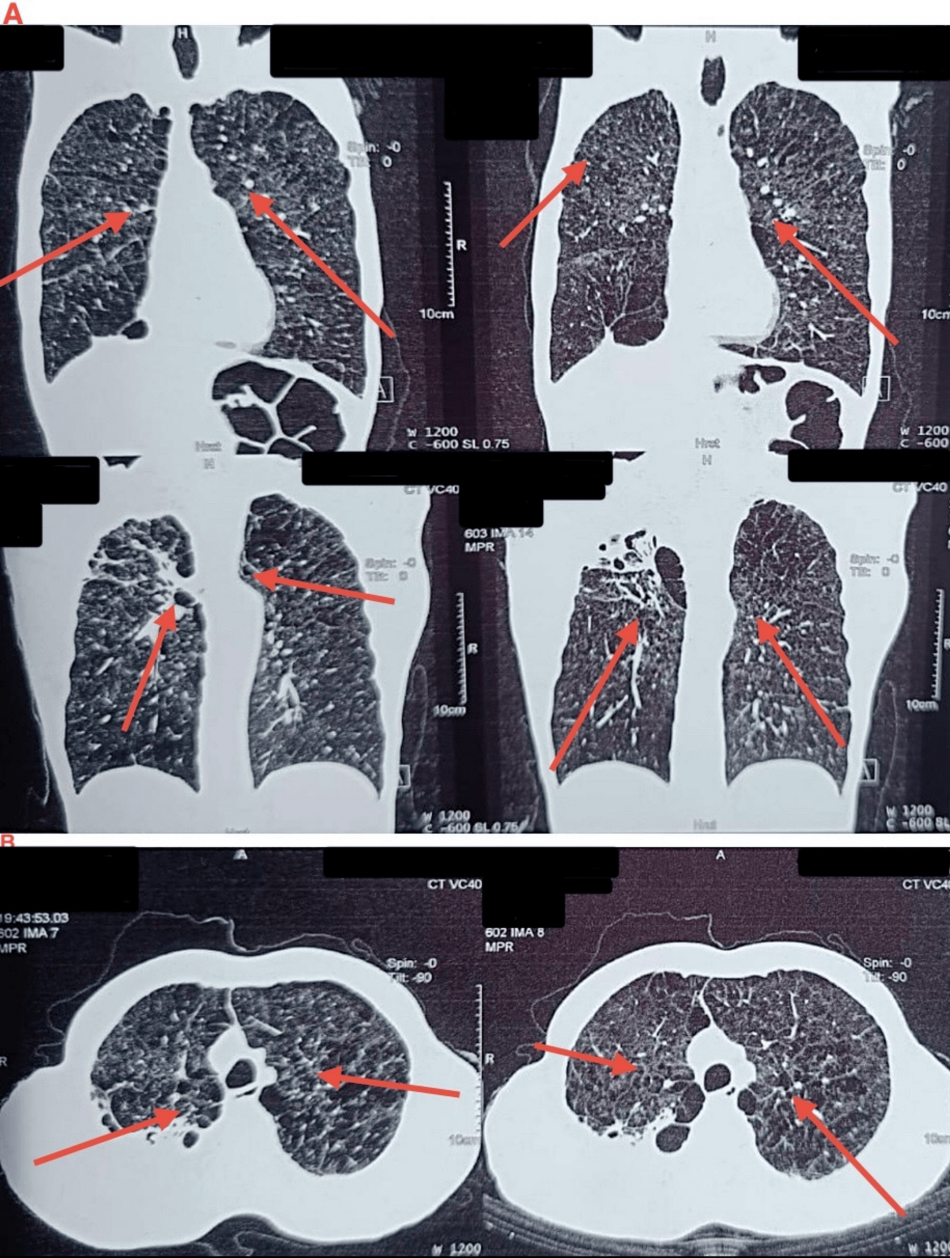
Contrast-enhanced CT of the thorax: (a) coronal section and (b) axial section, suggestive of bronchiectasis (red arrows).
